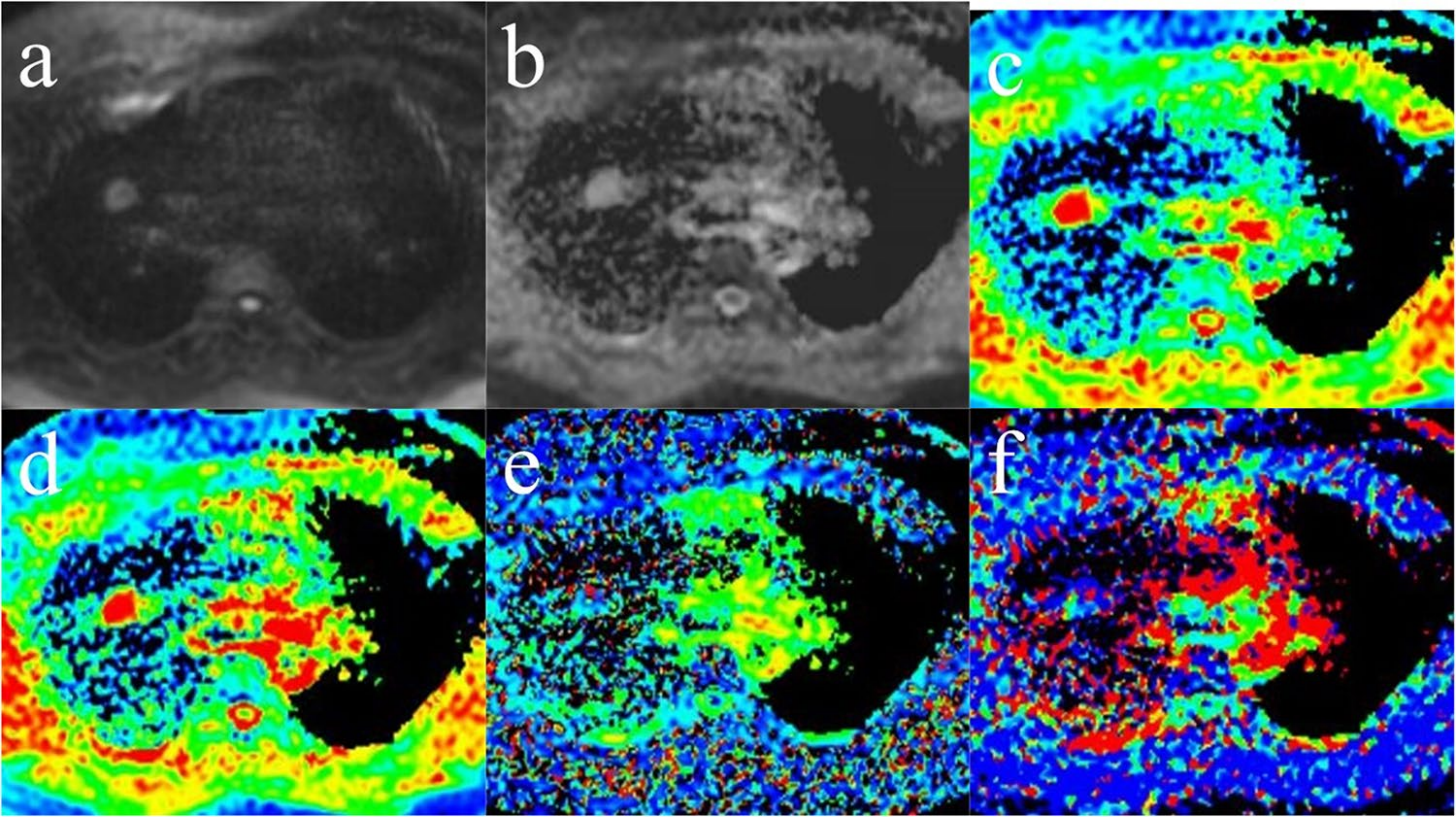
Pulmonary hamartoma in the right upper lobe. (a) Diffusion-weighted imaging (DWI) (*b* = 800 s/mm^2^); the signal intensity (SI) of the lesion was decreased, and the lesion-spinal signal intensity ratio (LSR) was 0.530. (b) ADC_800_ map. (c) ADC_total_ map. (d) Pure diffusion coefficient (D) map. (e) Perfusion fraction (f) map. (f) Perfusion-related diffusion coefficient (D_star_) map.
